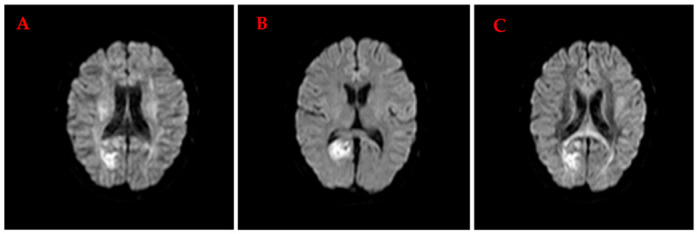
(A–C) Cerebral MRI T2-Weighted (T2W): Area of right parasagittal T2 hypersignal with recent ischemia (A). Cerebral MRI T2-Weighted (T2W): Right parasagittal parietal ischemic lesion B,C).
